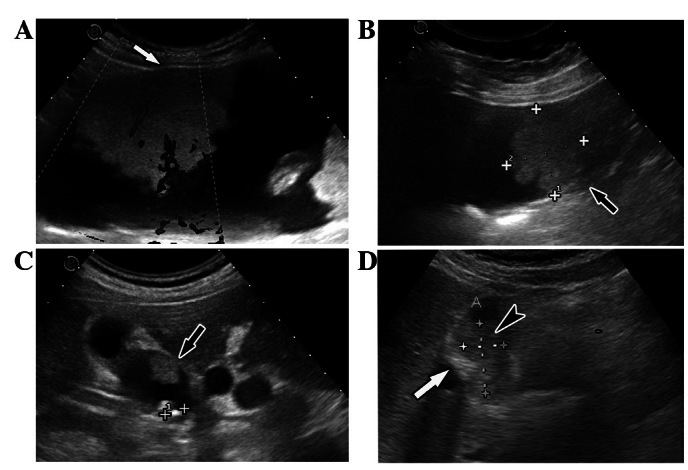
Ultrasonography (US) of adenomas of the gallbladder. The arrows show the wall of the gallbladders were thin and ran smoothly, and the demarcation between the wall and mass was clear. The black arrow in image (C) shows the mass had a narrow base and there was space between the mass and wall. (D) US of gallbladder carcinoma. The white arrow indicates the thickened wall and dotted lines indicate the size of the mass. The base was wide and the demarcation between the wall was obscure.
